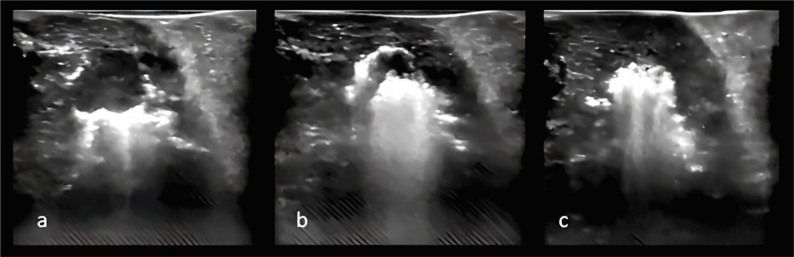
Intraoperative ultrasound (a, b and c).
